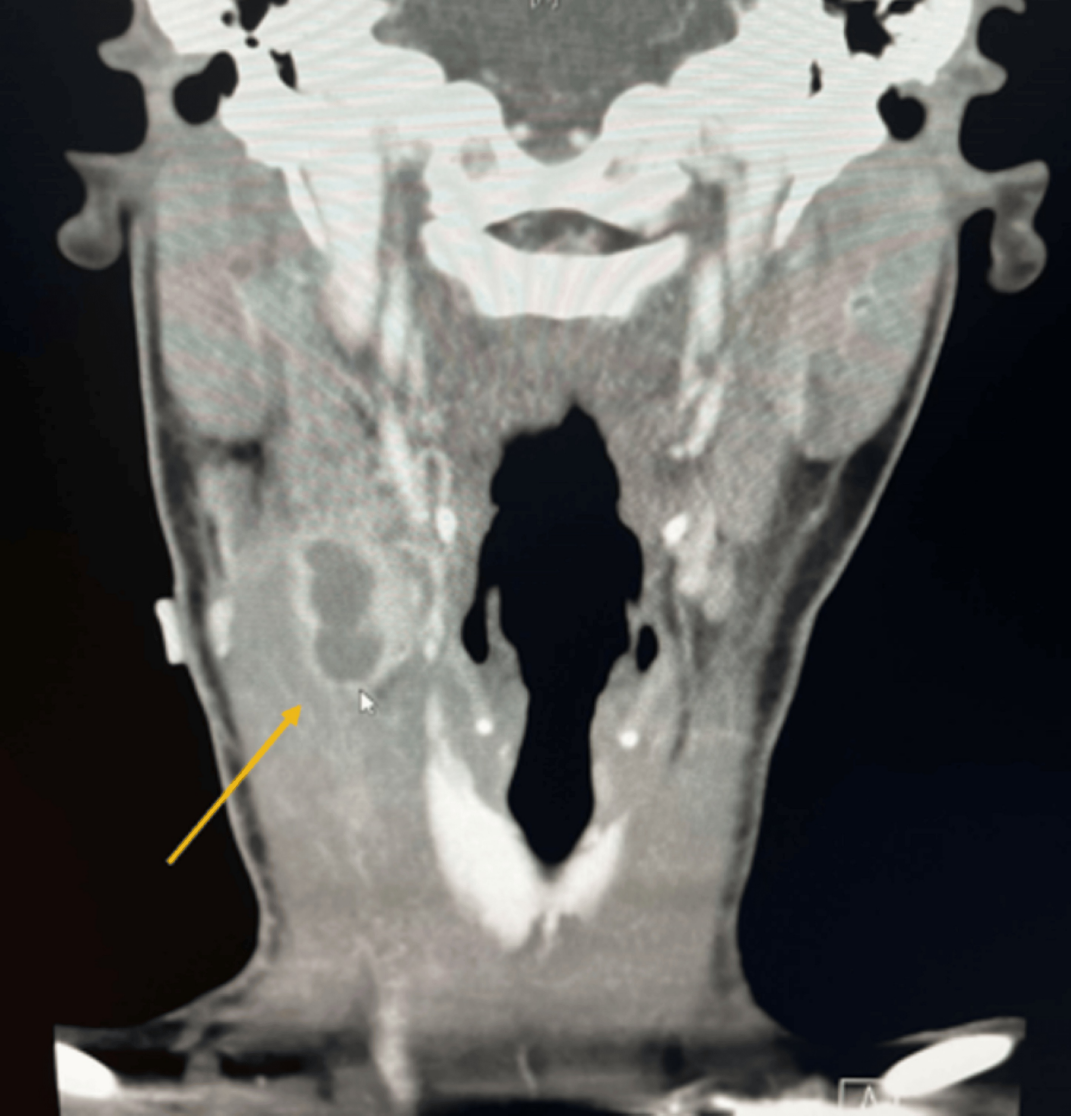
Follow-up CT imaging on day four showing a necrotic mass measuring 3.6 x 1.7 cm in the right cervical chain with adjacent sternocleidomastoid myositis and mass effect on the internal jugular vein.
